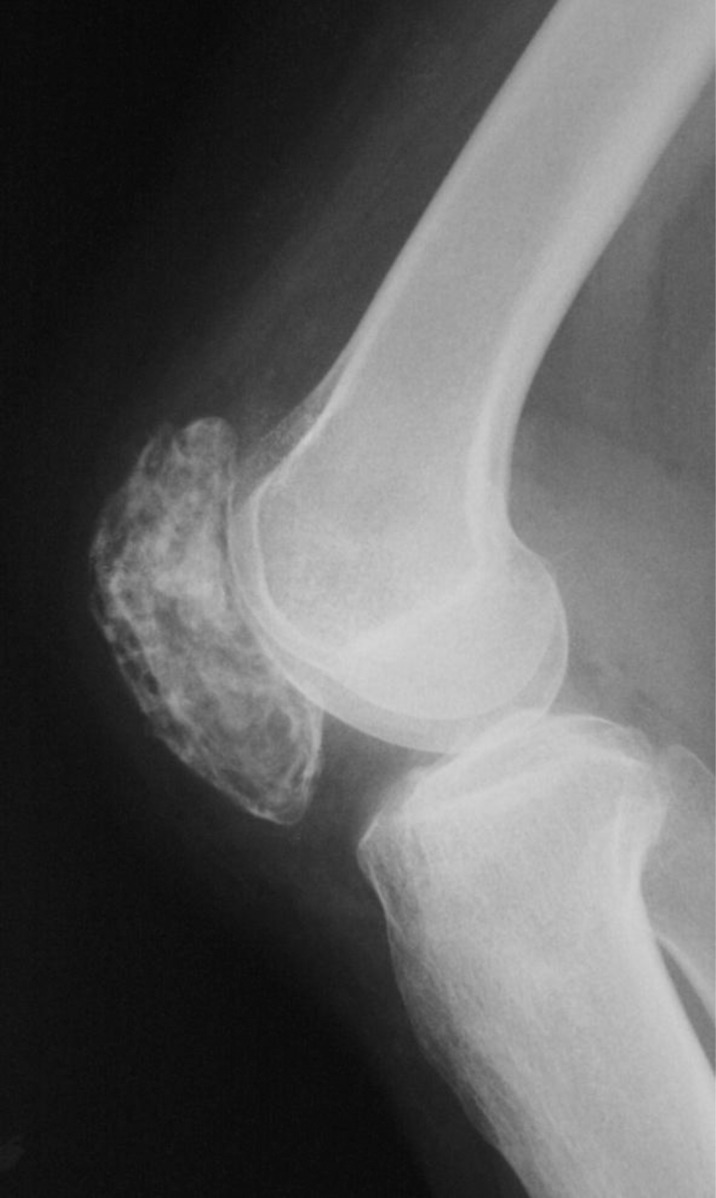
A lateral radiograph of the right knee. The right patella was enlarged, and its cortical shell was irregularly discontinued. Osteolytic and osteoblastic lesions were irregularly distributed in the patella.
